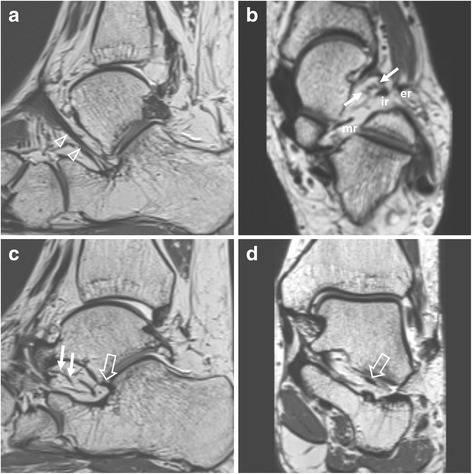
A 27-year-old woman with subtalar instability. Sagittal (a) and axial (b) isotropic 3D T2-weighted images demonstrating intact inferior extensor retinaculum (arrowheads). er, ir, and mr indicate external, intermediate, and medial root of inferior extensor retinaculum, respectively. Cervical ligament represents a bundle of fan-shaped striated fibers (solid arrows). Sagittal (c) and coronal (d) isotropic 3D T2-weighted images demonstrating a thin and narrow anterior capsular ligament (open arrows). Solid arrows indicate cervical ligament with thin sheet-like fan-shaped striped fiber bundles
